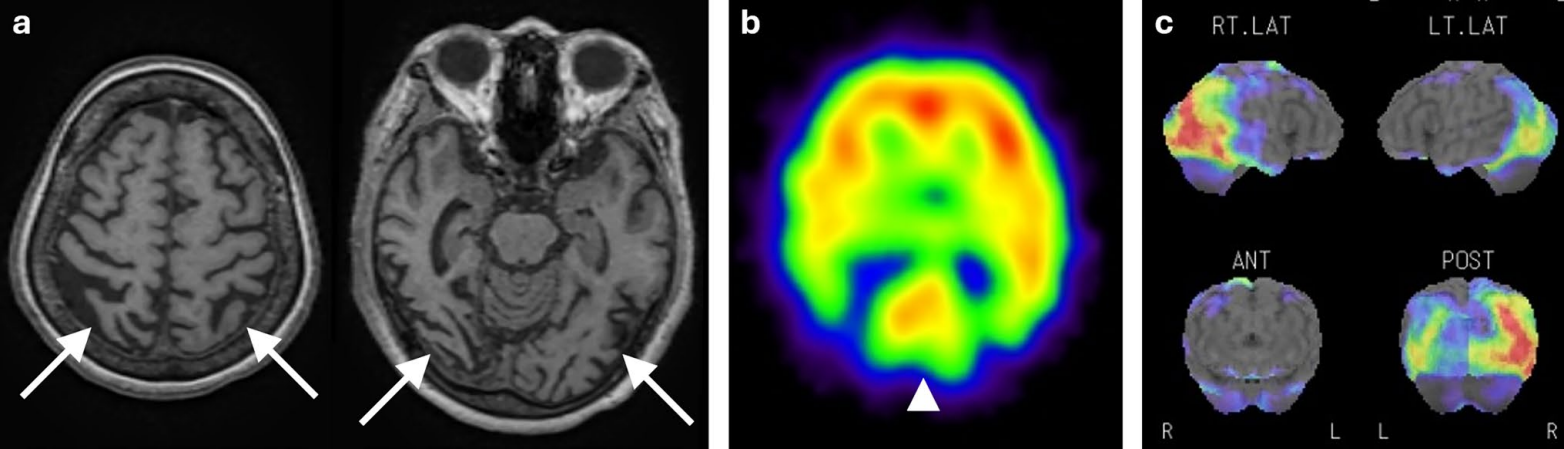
A 73-year-old female with posterior cortical atrophy with left hemispatial neglect, visual agnosia, alexia with agraphia, prosopagnosia, and simultanagnosia. Elevated CSF pTau and decreased CSF Aβ-42 levels indicated AD as background pathology. a T1-weighted image (T1WI) showed right-dominant atrophy in the parietal, occipital, and temporal lobes (arrows). b IMP brain perfusion images demonstrated a CIS-like finding (arrowhead). c 3D-SSP analysis revealed hypoperfusion consistent with atrophic regions. This case was presented in a previous study [31]
